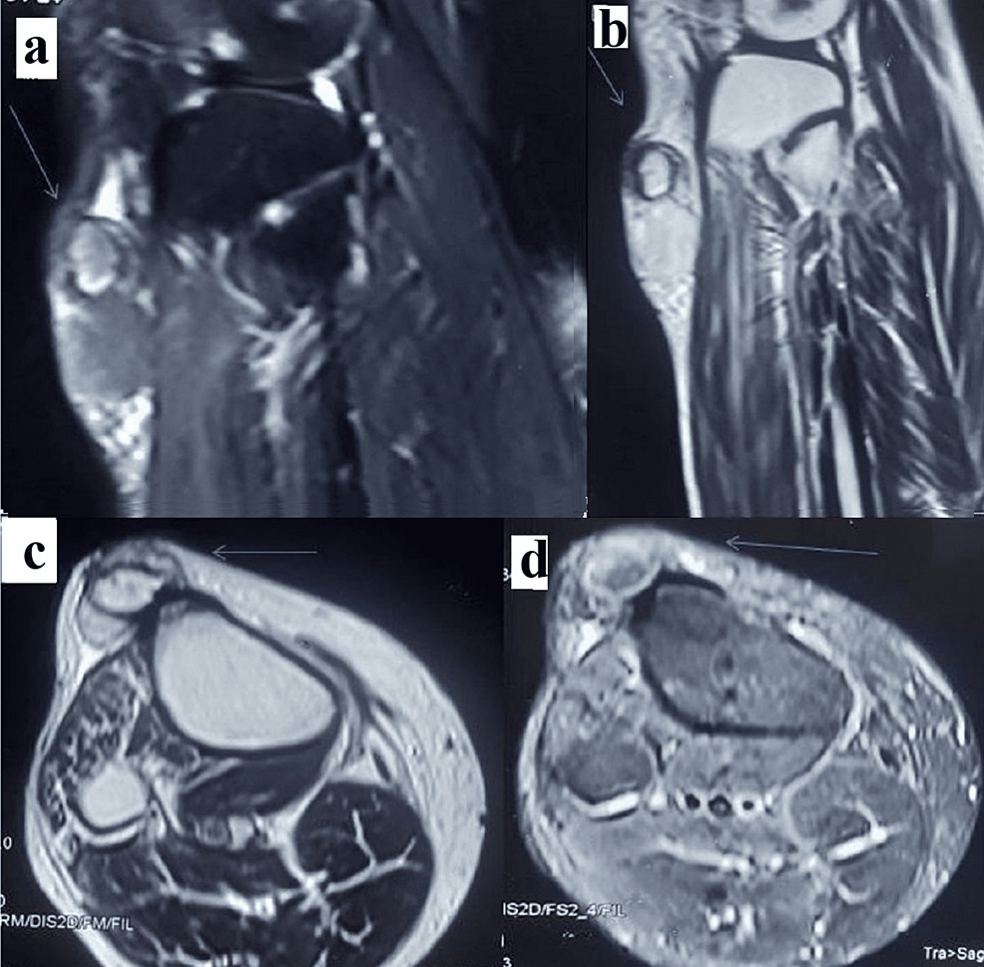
MRI knee MRI knee (with arrows depicting the lesion), (a) is a sagittal T2W image showing a hypo-intense lesion with a hyper-intense rim in the subcutaneous plane anterior to the tibial tuberosity. (b) and (c) are sagittal and axial images T1W images respectively which show hyperintense lesions. The same lesion (d) fat suppression in the STIR sequence suggests chronic ossified infrapatellar bursitis
